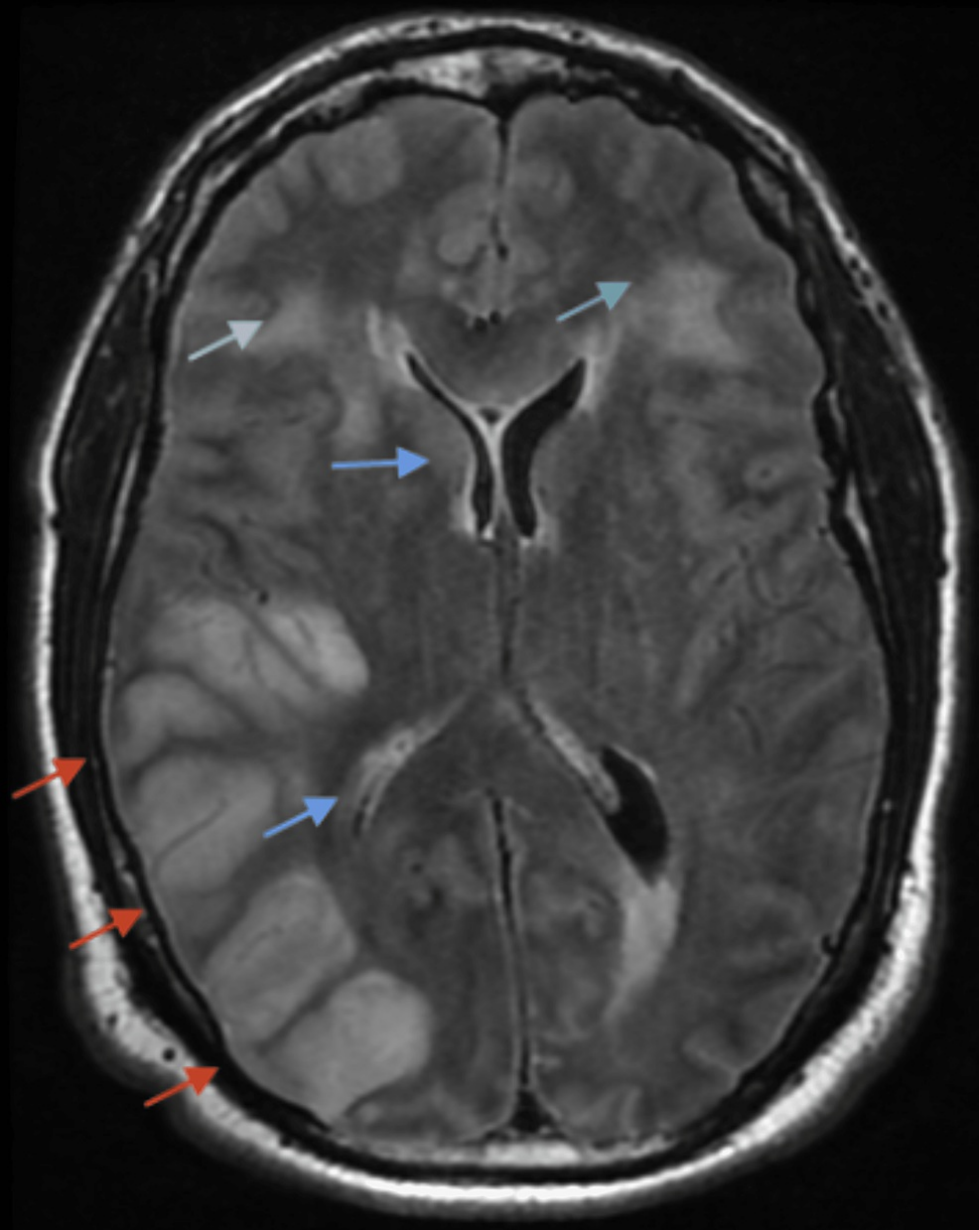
MRI brain FLAIR T2 weighted series showing diffuse sulcal edema in the temporal, parietal, occipital regions (red arrow), effacement of the right lateral ventricle (blue arrow), and bifrontal edema (green arrow)
